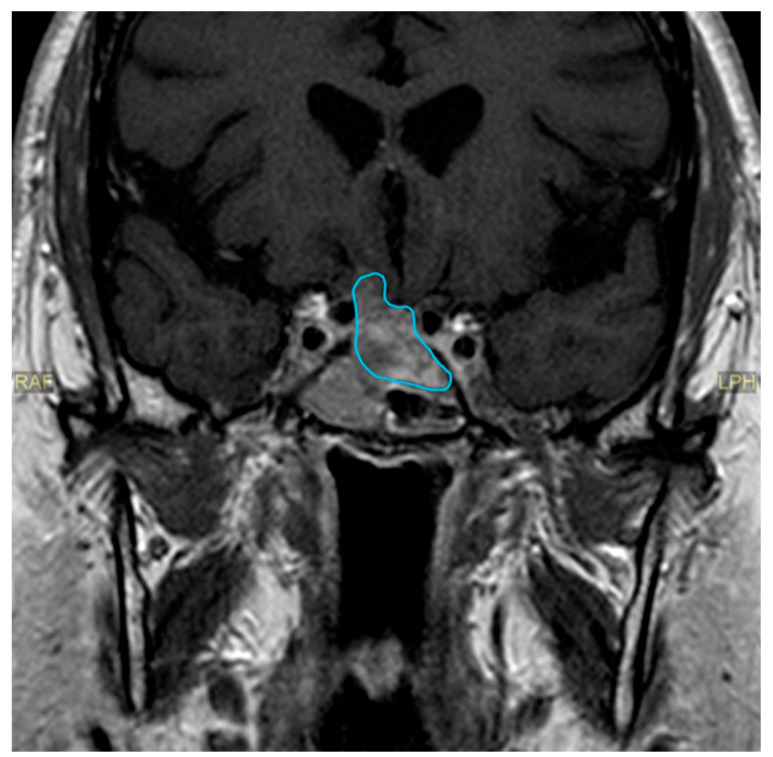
MRI, June 2020. Adenomatous recurrence with right optoquiasthmatic compressive effect, MRI, magnetic resonance imaging.
